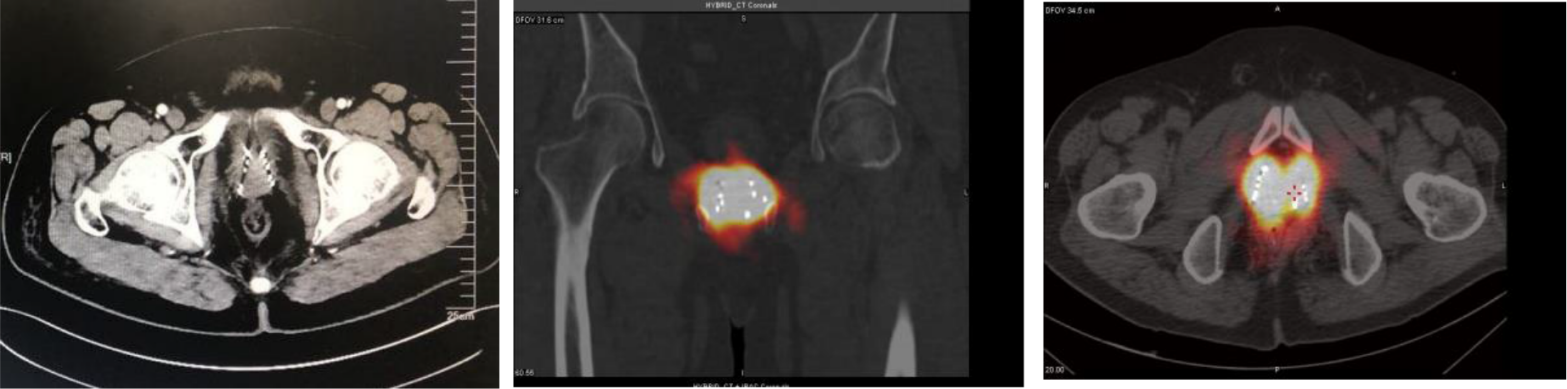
After 1 month of re-examination, no change was observed in tumor size, and SPECT showed that the radioactive concentration of particles basically covered the target area.
